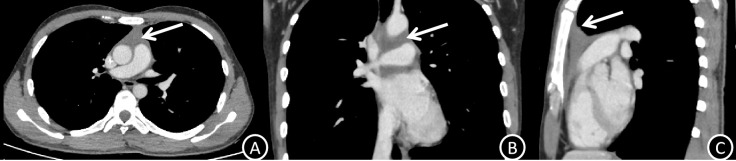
(A–C) Post-treatment venous phase CT images (axial, coronal, and sagittal planes) demonstrate marked regression of the anterior mediastinal mass (white arrows) compared with pre-treatment imaging, consistent with partial response to systemic therapy.
